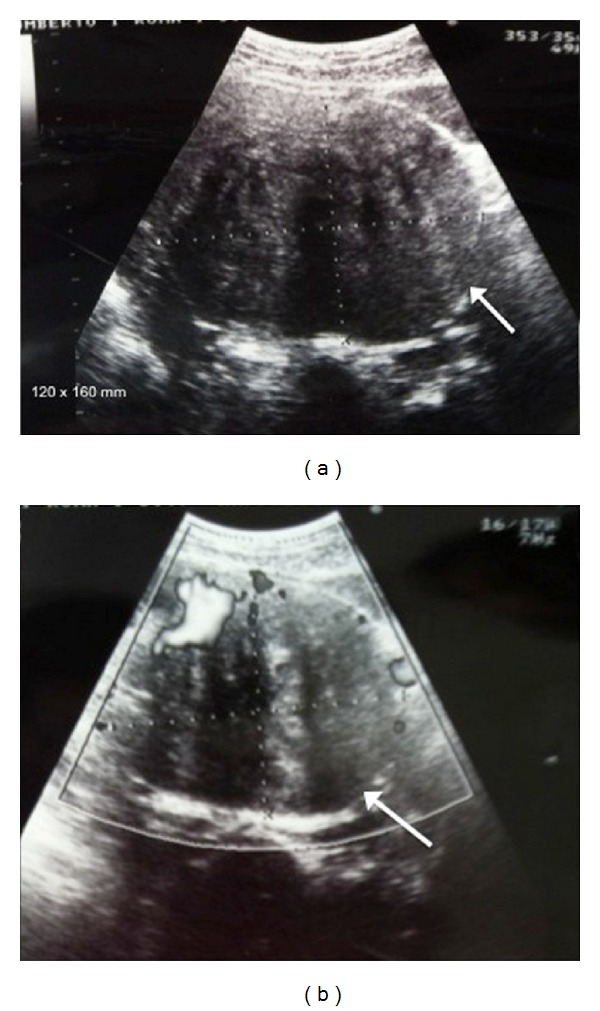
Myoma at ultrasonographic exam.
